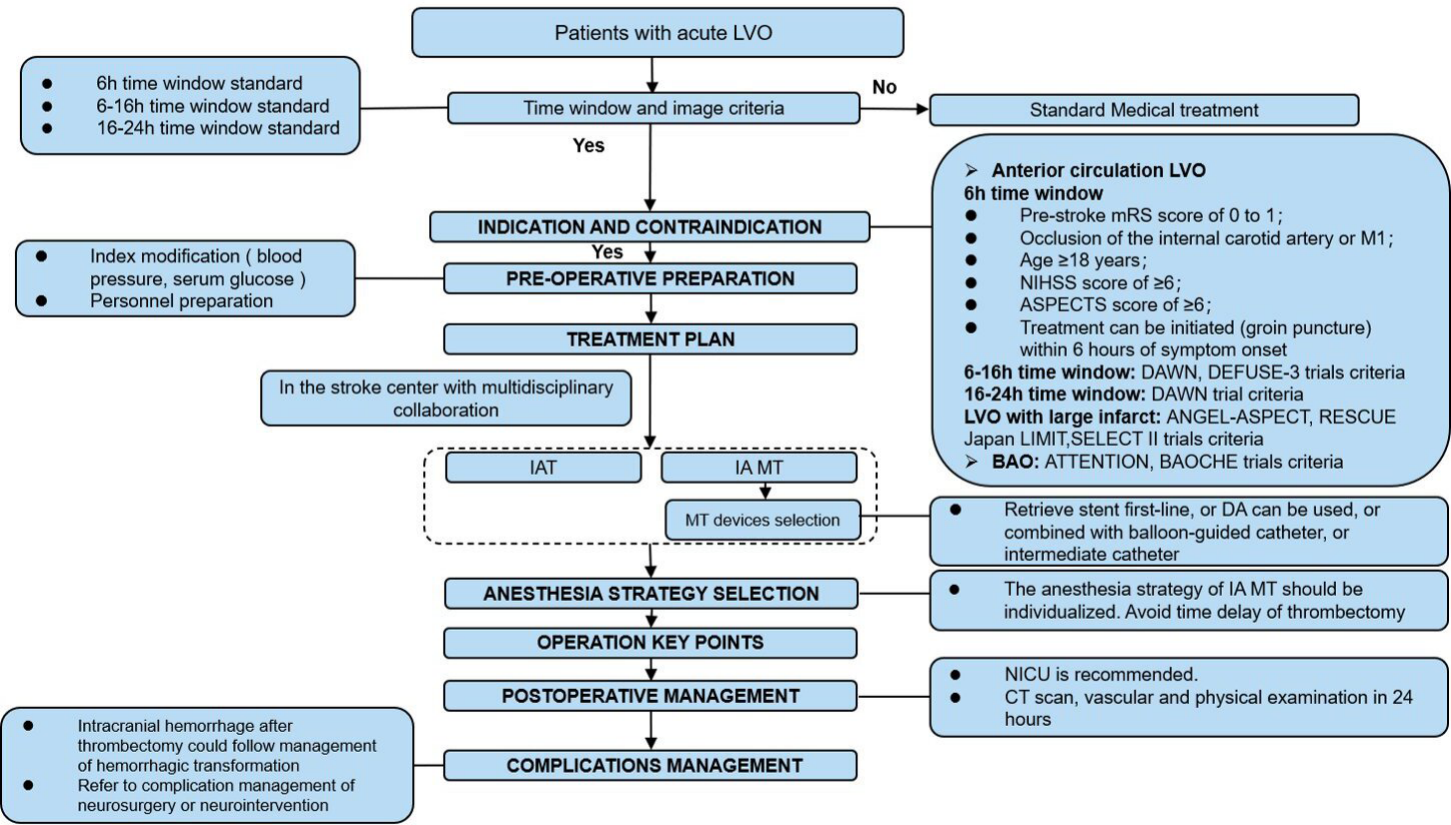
The intra-arterial (IA) mechanical thrombectomy (MT) for patients with acute ischaemic stroke. ASPECTS, Alberta Stroke Program Early CT Score; BAO, basilar artery occlusion; DA, direct aspiration; IAT, intra-arterial thrombolysis; IV, intravenous; LVO, large vessel occlusion; mRS, modified Rankin Scale; NICU, neurological intensive care unit; NIHSS, National Institutes of Health Stroke Scale.
